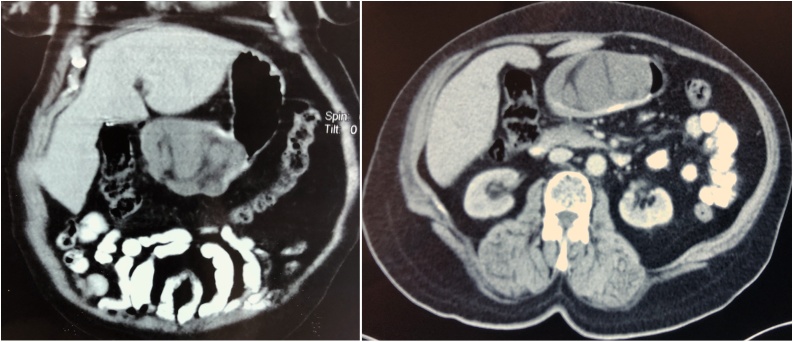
CT-scan of the abdomen showing evidence of a large non-enhancing intramural mass in the gastric wall with narrowing of the pyloric canal.
